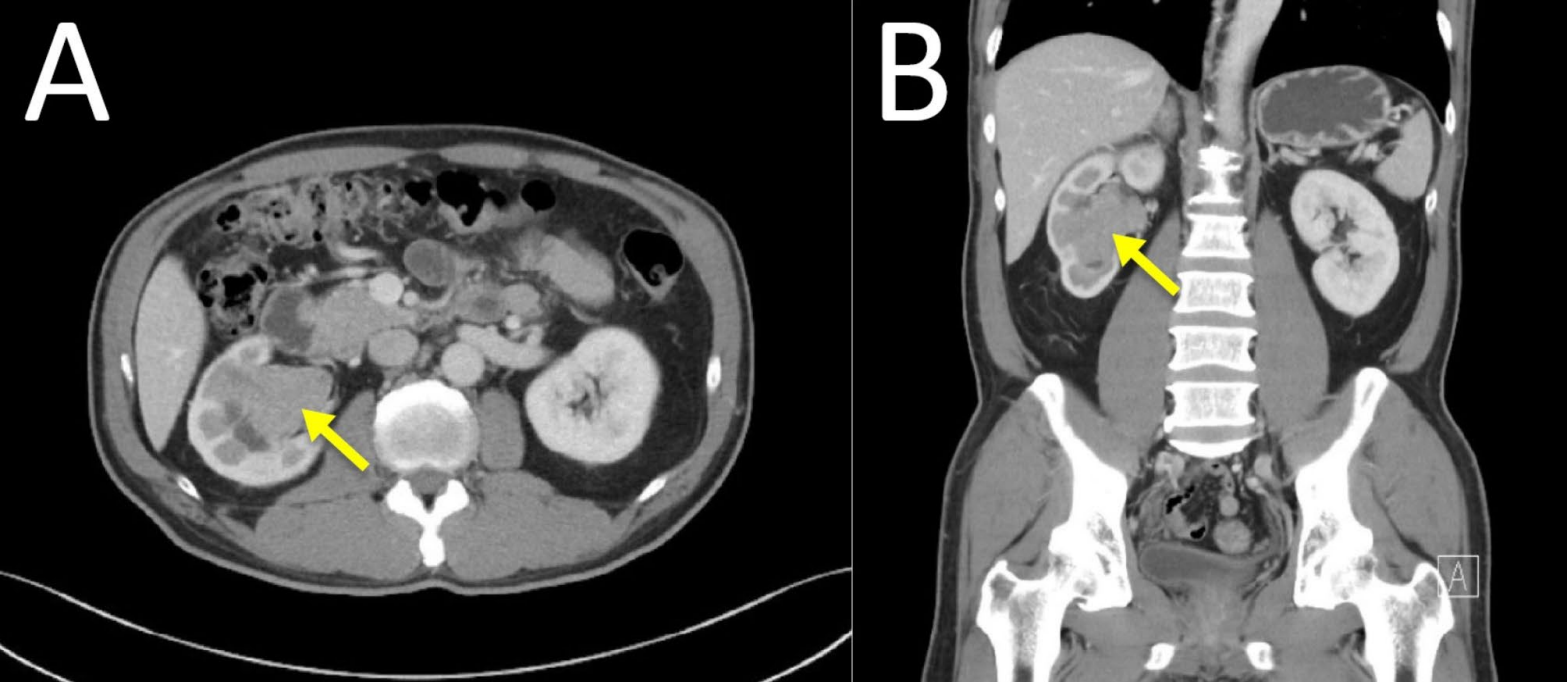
A, B: Axial and coronal views of the abdominal and pelvic computed tomography (CT) images demonstrate a preoperative enhancing tumoral lesion (arrow) measuring up to 5.75 cm in the right renal pelvis. The lesion involved the renal parenchyma and proximal ureter, with associated right hydronephrosis, indicating obstruction at this site
